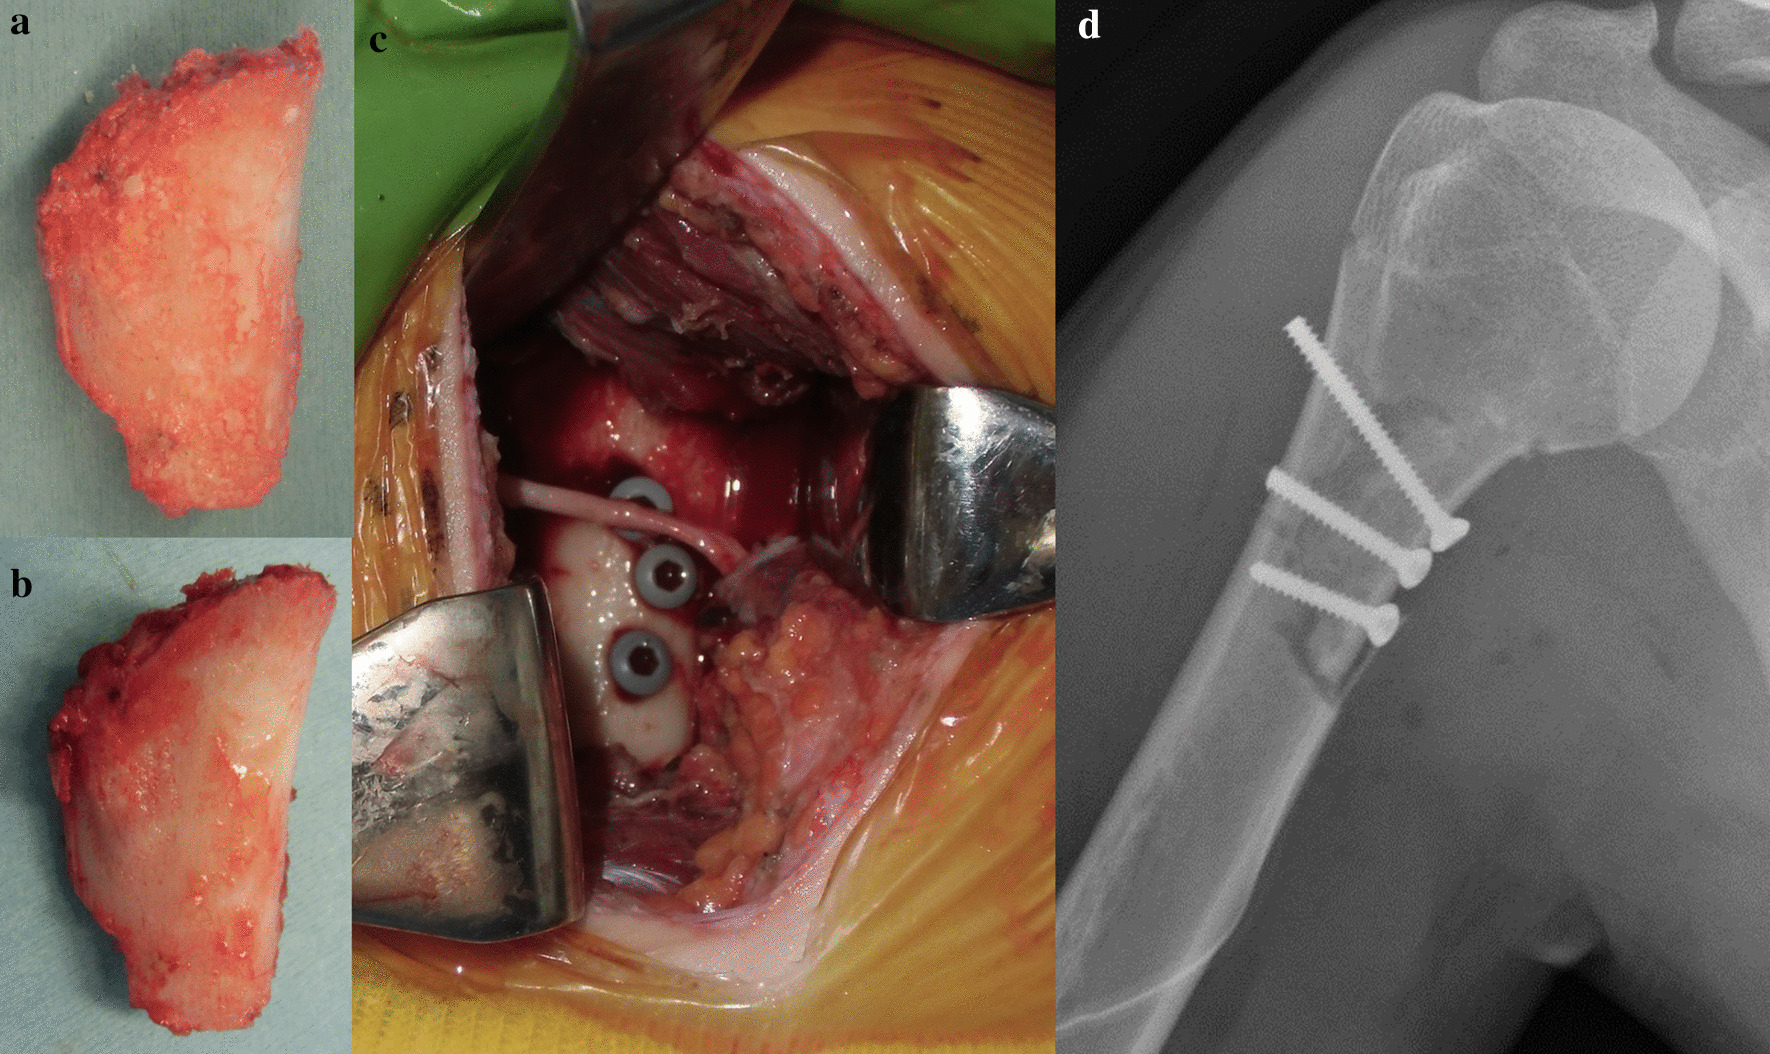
Surgical treatment for a proximal humeral lesion and the postoperative roentgenogram. a, b The retracted hemicortical bone of the proximal humeral lesion was treated with liquid nitrogen (a) and was dissolved at room temperature, and washed by 0.3% iodine saline and distilled water (b). c The frozen autograft was returned to the original position with screw fixation, preserving axillary nerve. d Postoperative roentgenogram was shown
